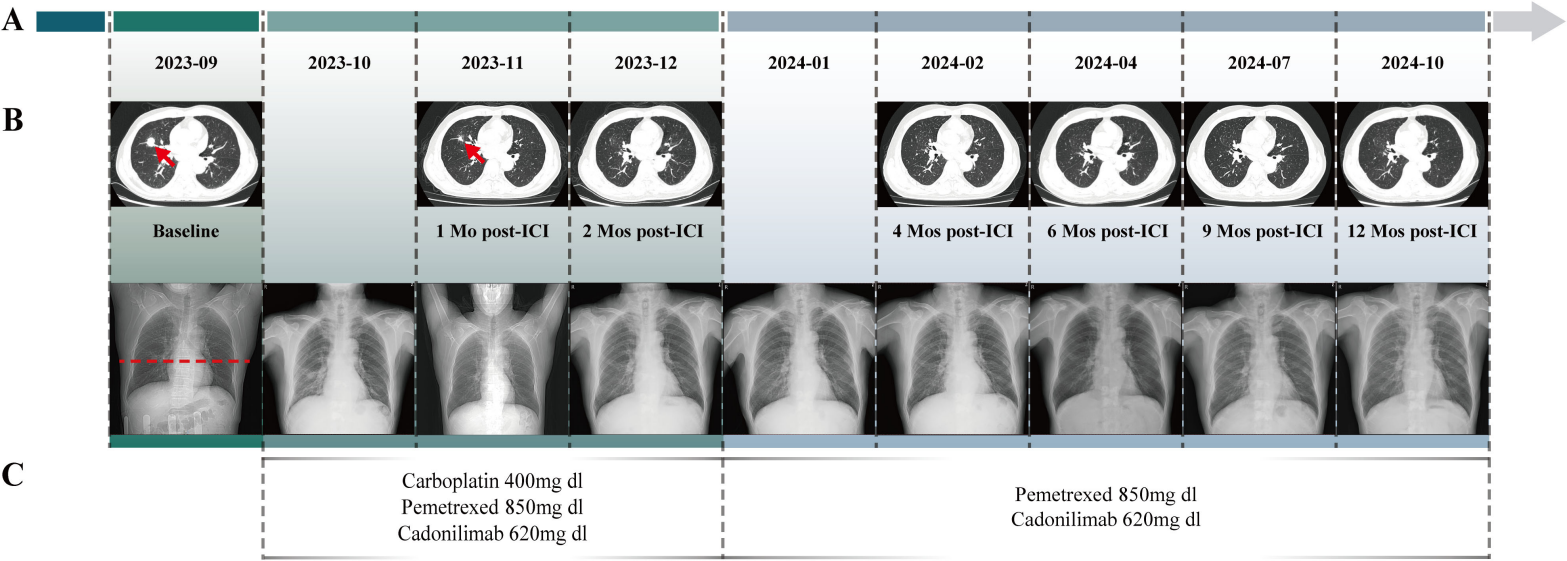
The Clinical Course of Lung Adenocarcinoma. (A) Timeline showing the clinical course. (B) Change of the tumor lesion revealed by radiological examinations (CT scans and chest X-rays). Radiological examinations demonstrated the progression of the lung adenocarcinoma. The baseline tumor on September 11, 2023, is marked by a red arrow, with its corresponding level on chest X-ray indicated by red dashed lines. The subsequent imaging on November 19, 2023 demonstrated a partial response with significant tumor shrinkage. Serial surveillance scans were performed, with the latest imaging obtained on October 21, 2024. (C) Timeline of the patient’s systemic treatment course. From October 8 to December 31, 2023, chemotherapy commenced with Pemetrexed and Carboplatin, along with the immunotherapy agent Cadonilimab. Since January 22, 2024, subsequent chemotherapy cycles with Pemetrexed and Cadonilimab were administered.
